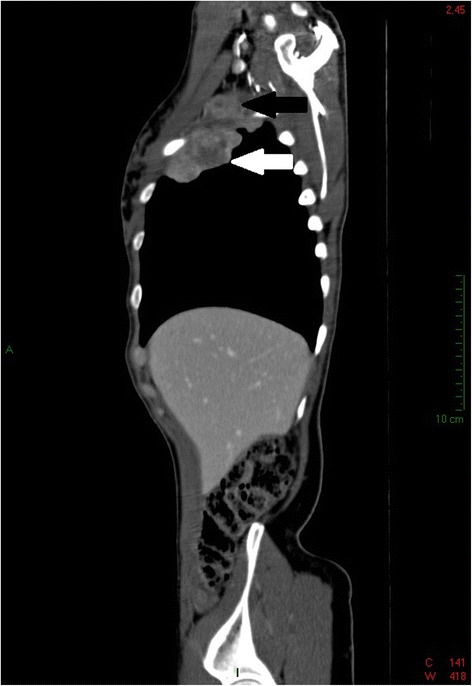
CT of the chest (96 × 96 dpi). Sagittal reformatted CT image demonstrates the presence of a parenchymatous neoformation (6 cm) in the right parietal pleura (white arrow) and of another mass in the right axillary extension (3 cm) (black arrow).
